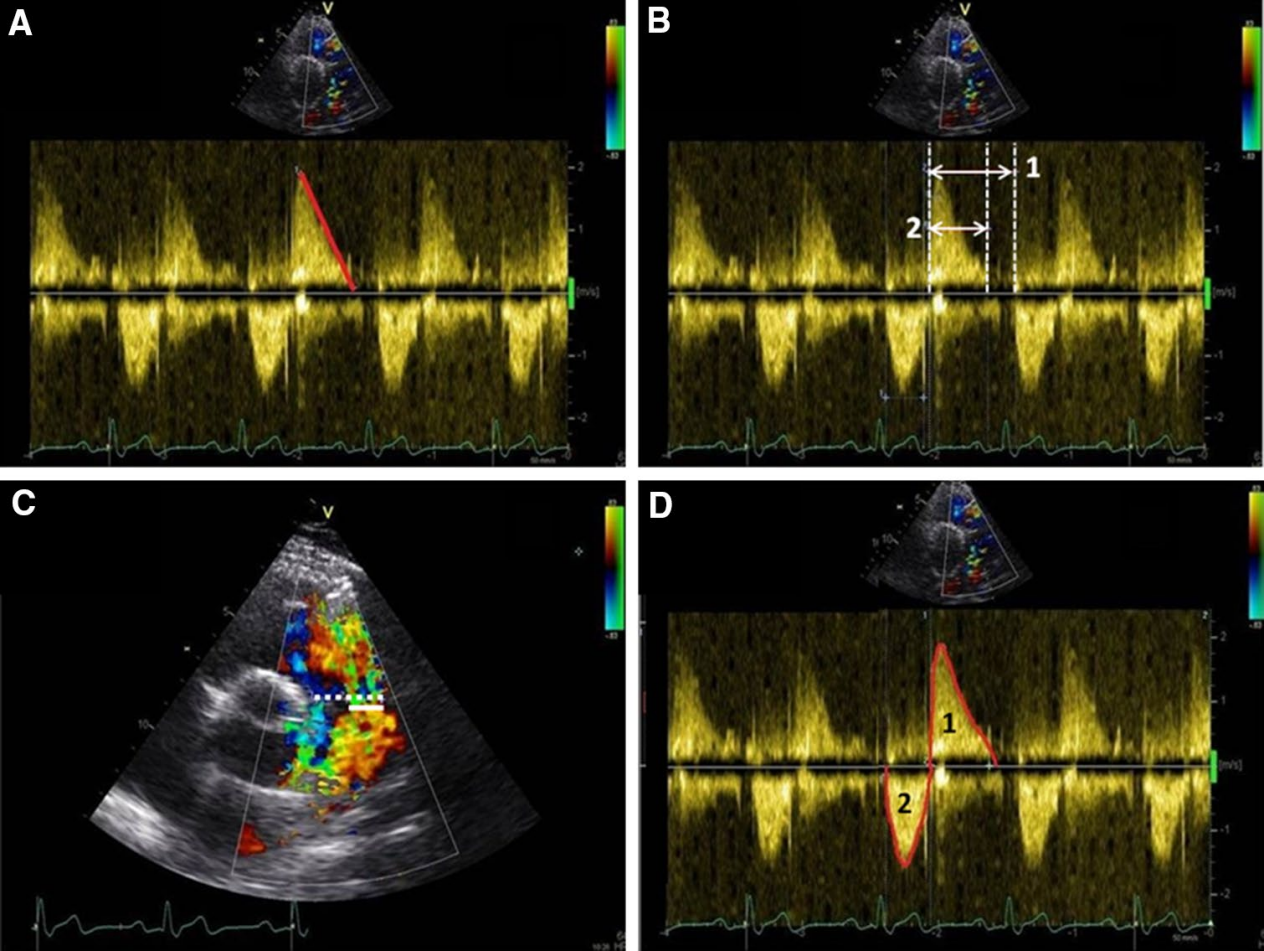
Doppler echocardiographic parameters. a PHT derived from CW Doppler echocardiography tracings. The deceleration slope of the PR (*red line*) represents the PHT. The PHT in this example is 118 ms, which suggests mild PR. b PRi measured with CW Doppler echocardiography. PRi is defined as the ratio of the duration of the regurgitation flow represented by *line 2* (in this example 433 ms) divided by the total diastolic time, *line 1* (in this example 646 ms). c The ratio of regurgitation jet width at the level of the PV (*straight line*) to PV annulus width (*dashed line*) measured during early diastole obtained by color-flow Doppler echocardiography. d DSTVI measured from the main pulmonary artery PW Doppler spectral tracing (*red lines*), is the area surface of the diastolic regurgitation flow (i.e. surface area 2) divided by the area surface of the systolic flow (i.e. surface area 1)
